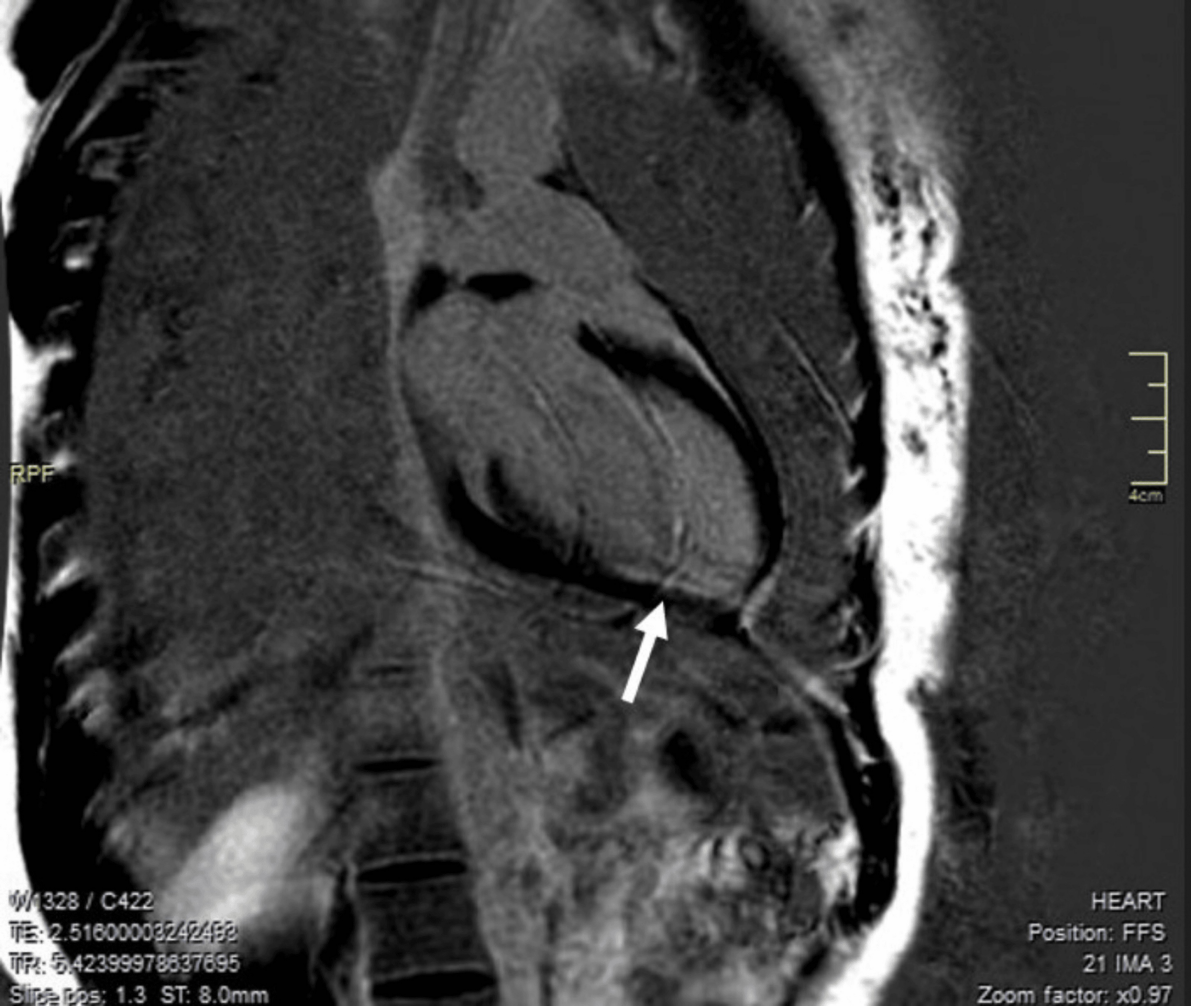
Cardiac MRI showing scaring suggestive of a small myocardial infarction (white arrow) MRI: magnetic resonance imaging
